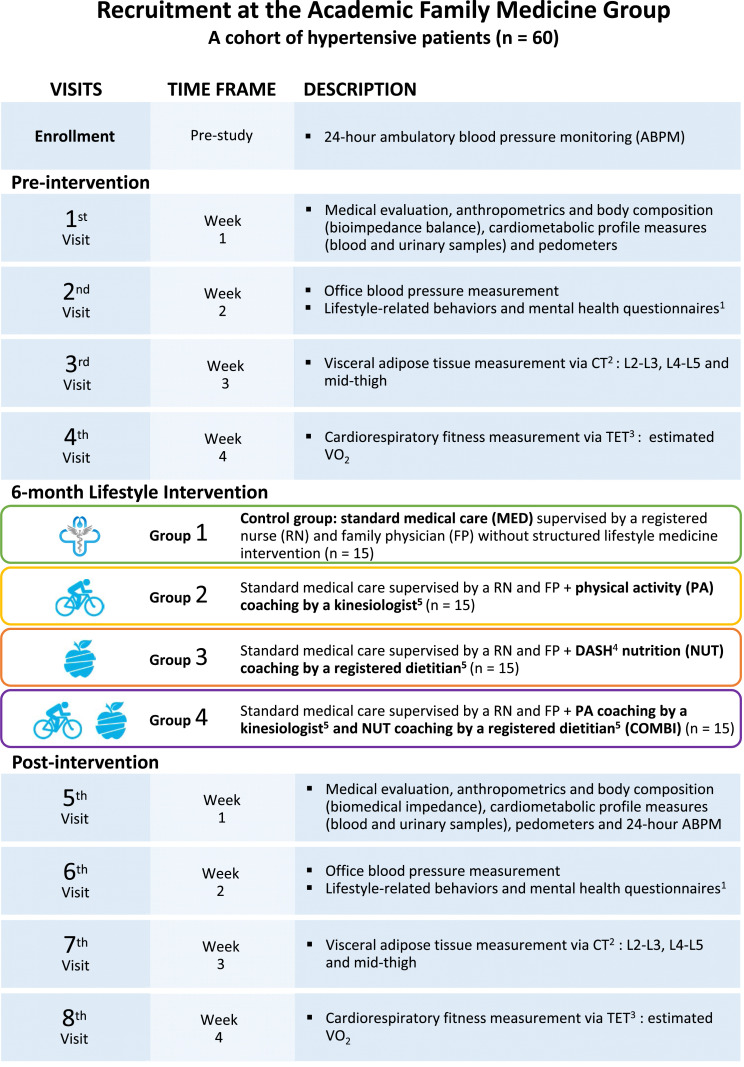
Study design and intervention details. Note: ^1^Questionnaires were Educoeur-en-route-77Q; physical activity assessment (3-day physical record); dietary assessment (3-day dietary record, Nutrition habit-27Q; Three-factor eating questionnaire-51Q (TFEQ), Beck Anxiety Inventory-21Q (BDI), State-trait Anxiety Inventory-20Q (STAI), SF-36-Q11 (quality of life); Food Frequency Questionnaire. Abbreviations: ^2^CT, computed tomography; ^3^TET, treadmill exercise test; ^4^DASH, Dietary Approaches to Stop Hypertension. ^5^Lifestyle intervention: all participants were meeting with a kinesiologist and/or a registered dietitian for 7 visits over 6 months, that is, 2 visits in the first month following by monthly visits for the 5 other months.
